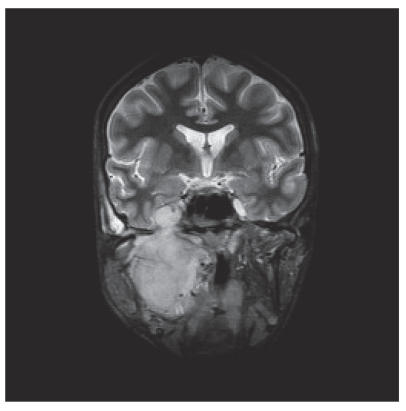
MRI (coronal view) showed the tumor in the right
